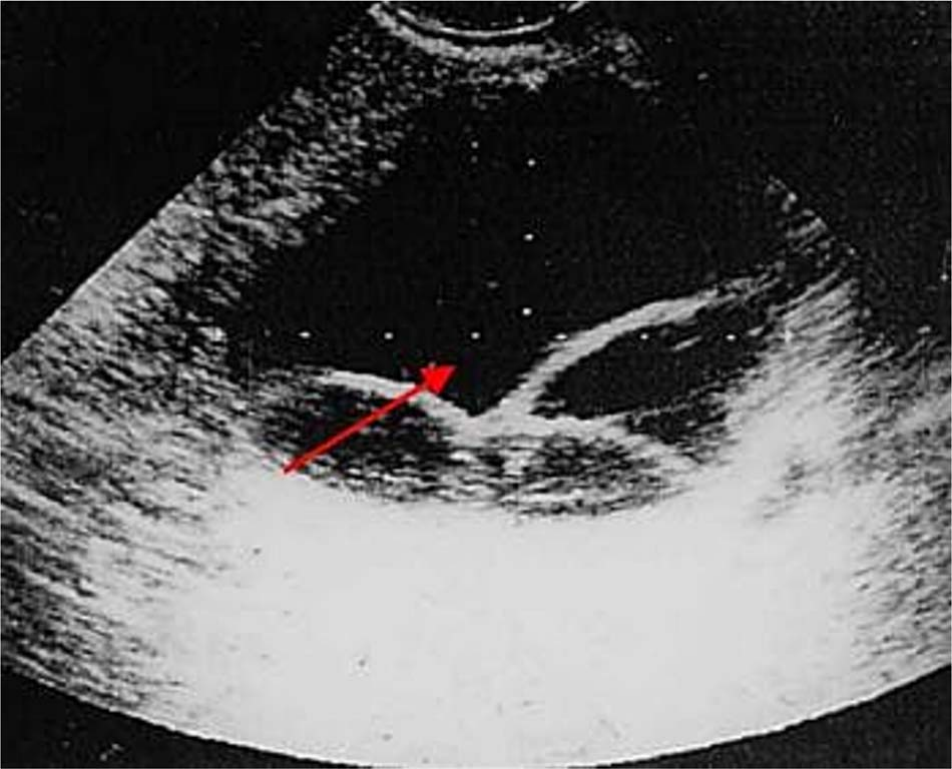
Ultrasound view of ovary of patient whit pelvic HC in the northern Iran.
